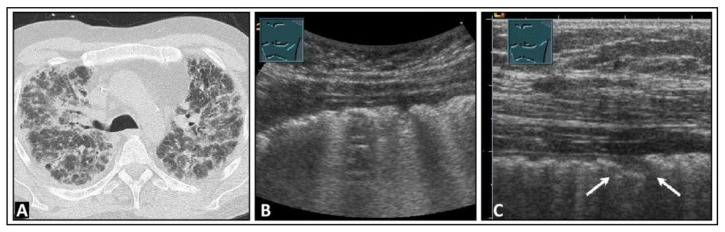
A patient with dyspnea and known advanced pulmonary fibrosis due to graft-versus-host disease following allogeneic stem cell transplantation. (A) The chest computed tomography scan displays severe fibrotic parenchymal changes. (B) On low-frequency lung ultrasound, multiple B-lines are observed on the left side, with a rough lung surface. (C) On high-frequency lung ultrasound, nodules up to 5 mm in size are observed (arrows).
